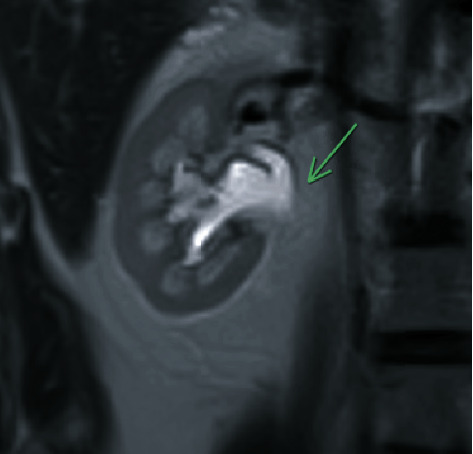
MRI shows a dilated right proximal ureter and pelvicalyceal system.
